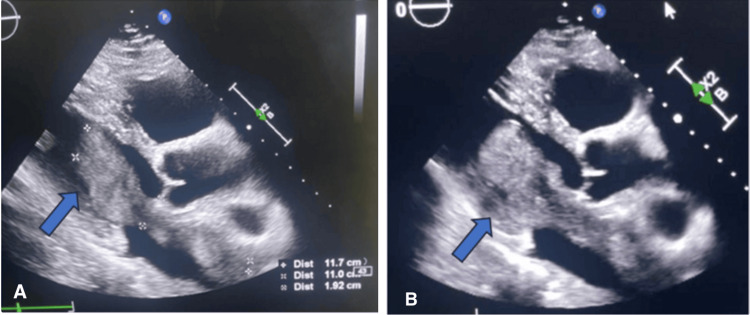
Parasternal long-axis section demonstrating the left atrium mass prolapsing into the left ventricle during diastole measuring 192 × 31 mm (arrow). (A) Parasternal long-axis section demonstrating the dimensions of the left atrial mass prolapsing into the left ventricle. (B) Ventricular implantation of the mass originating from the left atrium.
